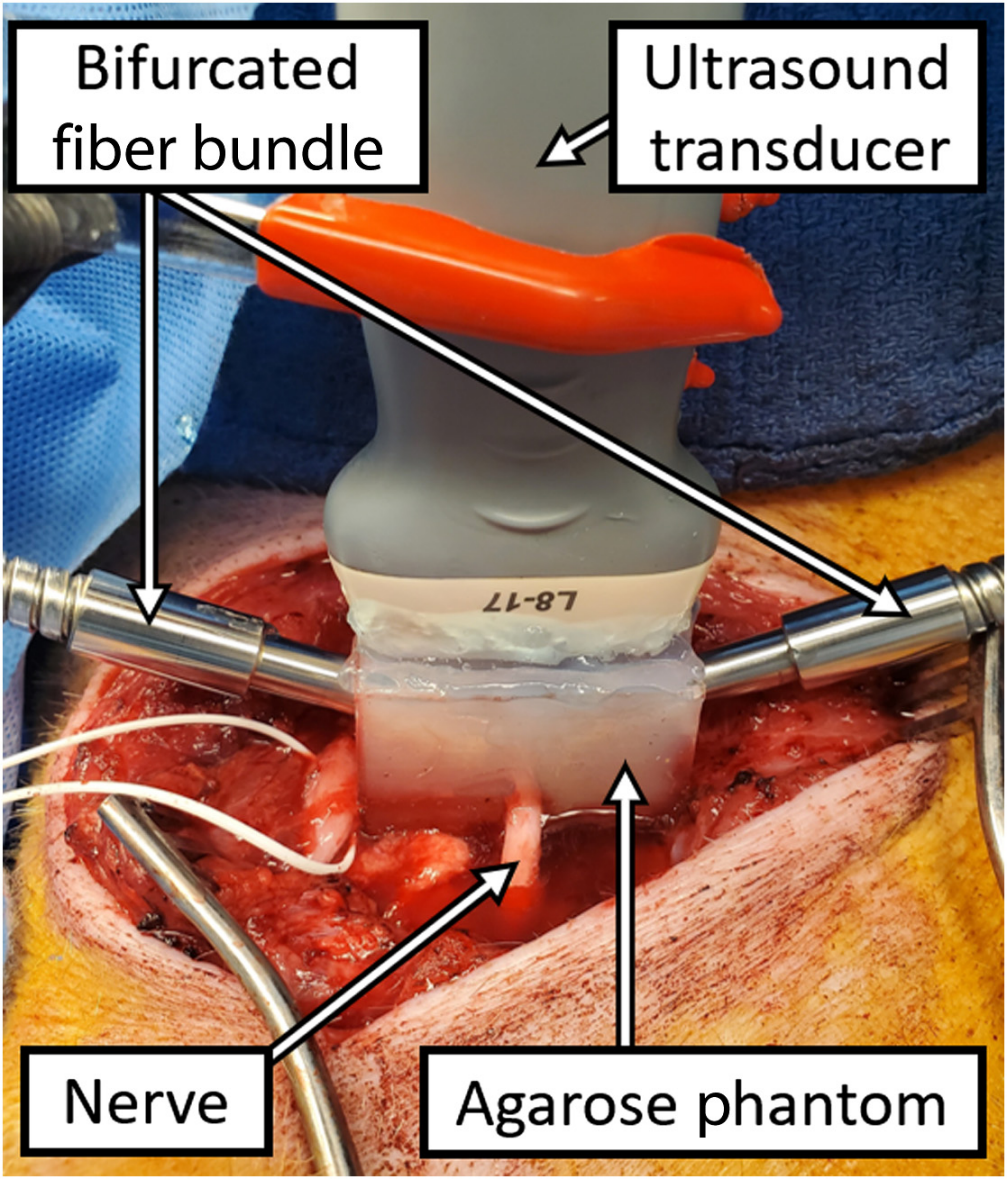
Experimental setup for *in vivo* photoacoustic imaging, including bifurcated fiber bundles secured in a custom-molded agarose block to bilaterally illuminate the nerve, with an ultrasound transducer positioned to image a circular cross section of the illuminated nerve.
